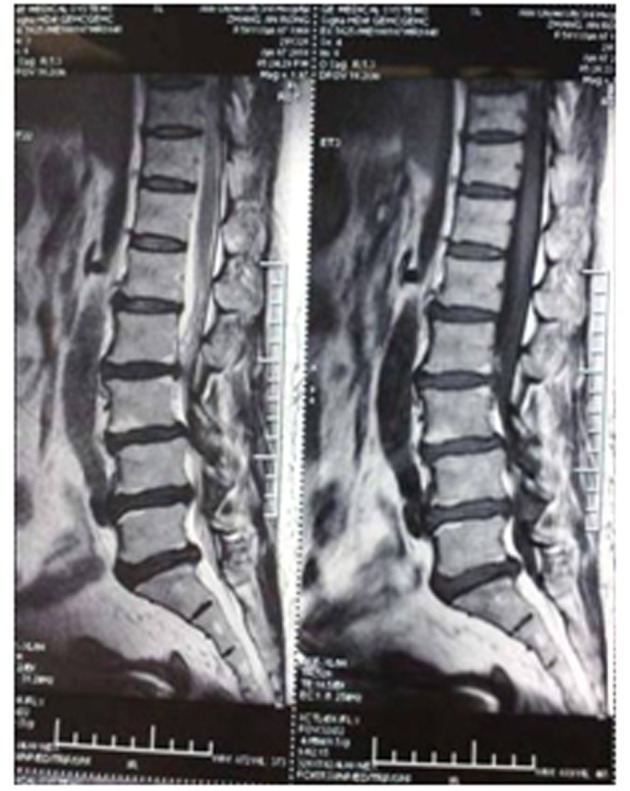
MRI revealed L3 spondylolisthesis, L45 and L5S1 disc herniation and spinal stenosis.
